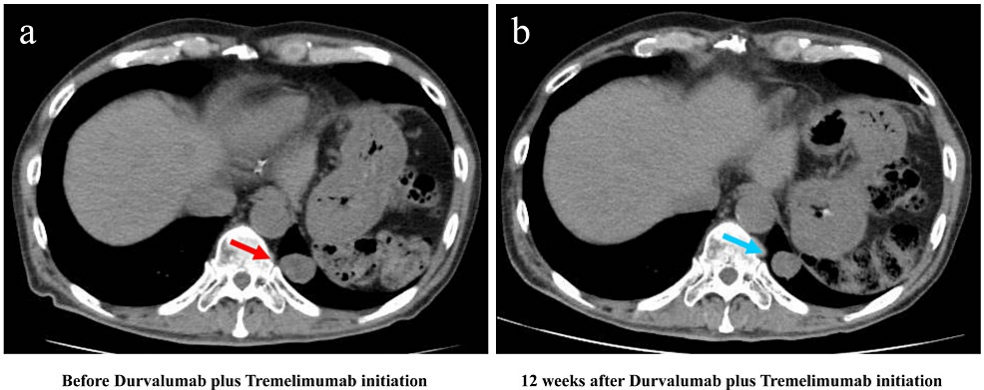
Image changes in CT before and after durvalumab plus tremelimumab initiation in Case 2 (a) CT image before durvalumab plus tremelimumab initiation (red arrow indicates lung metastasis); (b) CT image 12 weeks after durvalumab plus tremelimumab initiation (blue arrow indicates slightly shrunken lung metastasis)
